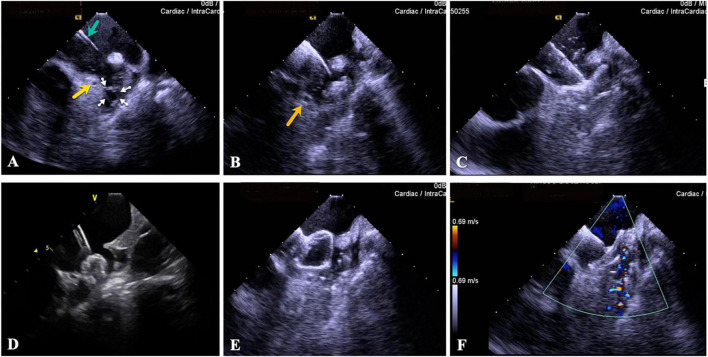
Process of ICE-guided LAAC. (A) Send the delivery sheath to the ostium of LAA (green arrow), and the pigtail catheter to the inside of LAA (small white arrow), and note the position of the LCX (yellow arrow). (B) Expand the closure device fixation disk, and note that it should be medial to the LCX (orange arrow). (C) Expand the closure disk. (D) Slightly withdraw the closure device (disk). (E) Pull test. (F) There is no residual shunt at the edge of the closure device as assessed by color Doppler flow monitoring at the end of closure. ICE, intracardiac echocardiography; LAA, left atrial appendage; LCX, left circumflex artery.
